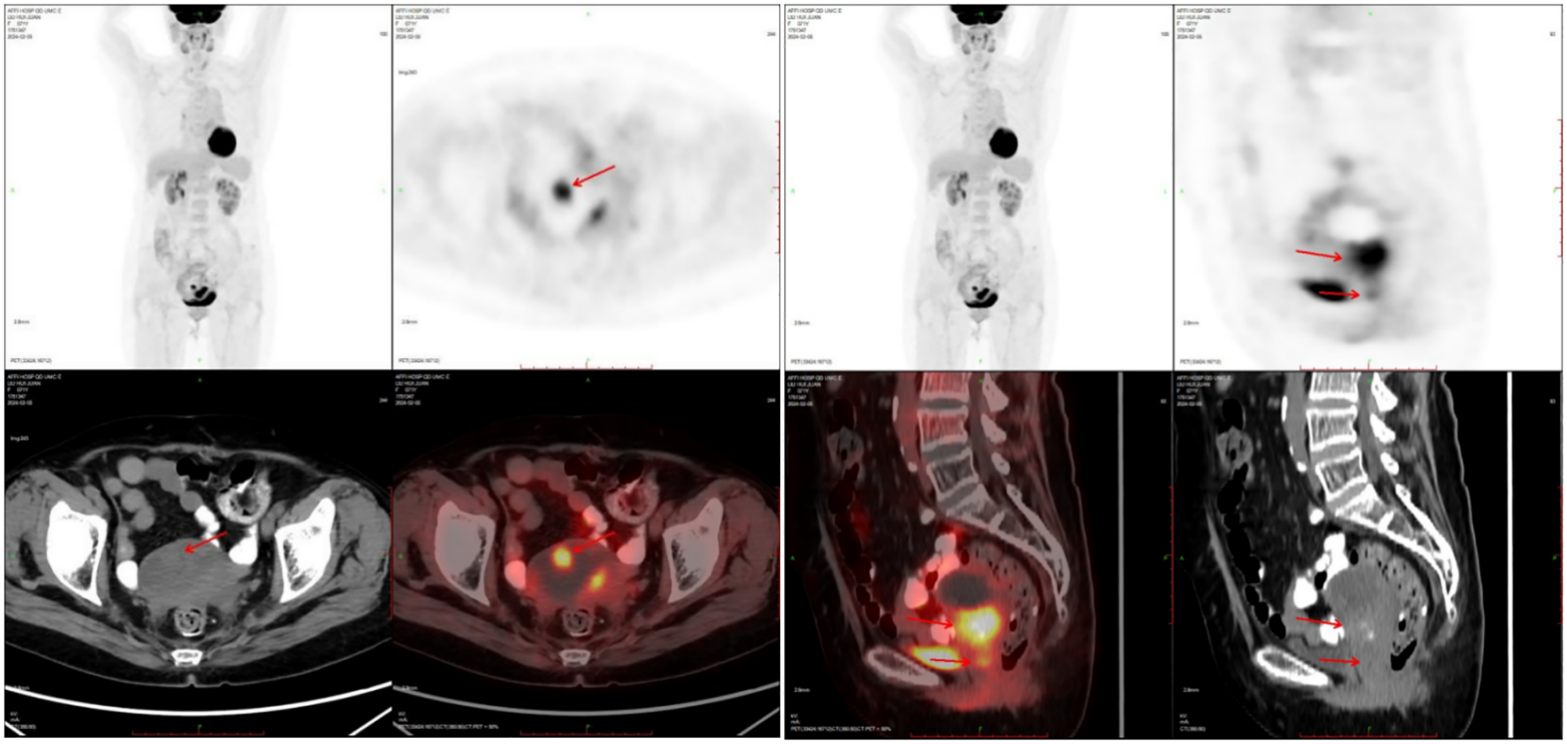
PET-CT showed nodules of soft tissue density at the base of the uterine body, protruding intrauterine, with increased metabolism, which was consistent with malignant uterine tumors.
